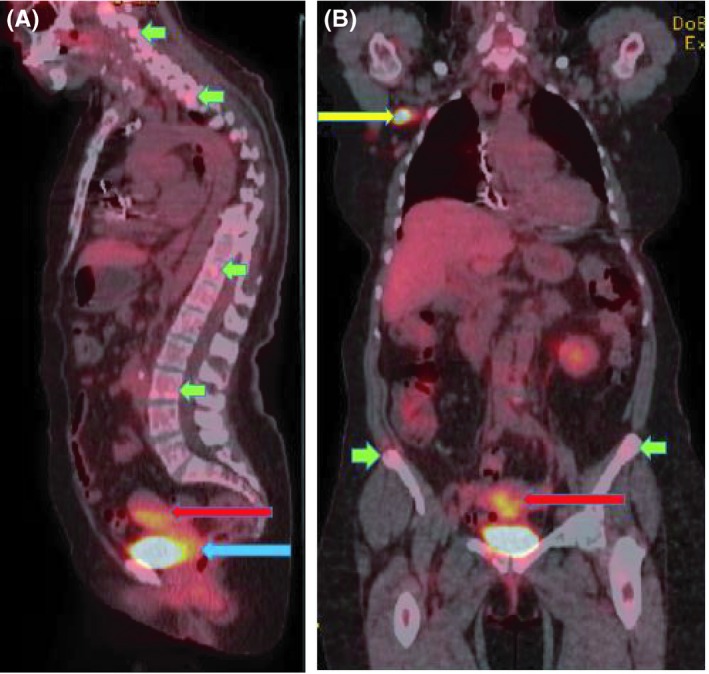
A, Coronal maximum intensity projection (MIP) image of a PET‐CT scan shows hypermetabolic activity in the cervix (blue arrow) and uterus (red arrow), with a maximum SUV of 4.9 (A). B, A right axillary lymph node measuring 2.6 × 1.5 cm (yellow arrow), with a maximum SUV of 12.7 is seen (B). Also present are multiple focal areas of uptake throughout the skeletal system (green arrows), predominantly at the level of the spine and bilateral iliac fossa, consistent with skeletal metastatic disease (A, B)
